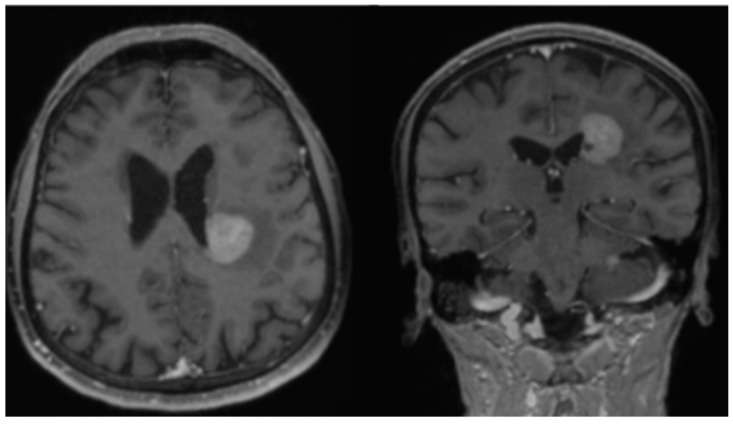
Brain MRI in January 2020, showing an enhancing mass on post-contrast T1 images.
